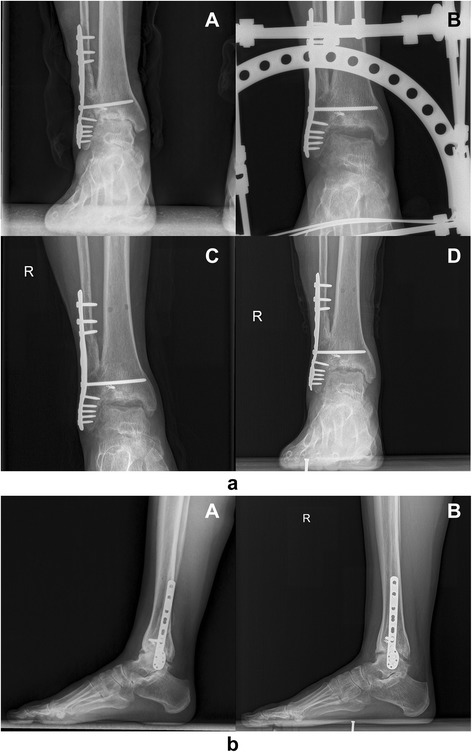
a radiographs of a female with severe ankle arthritis. *a* preoperative ankle joint. *b* ankle joint during ankle distraction. *c* ankle joint after removal of external fixation. *d* 1 year postoperative. b lateral radiographs of a female with severe ankle arthritis. *a* lateral view of the same patient before operation. *b* lateral view of the same patient at 1 year after operation
